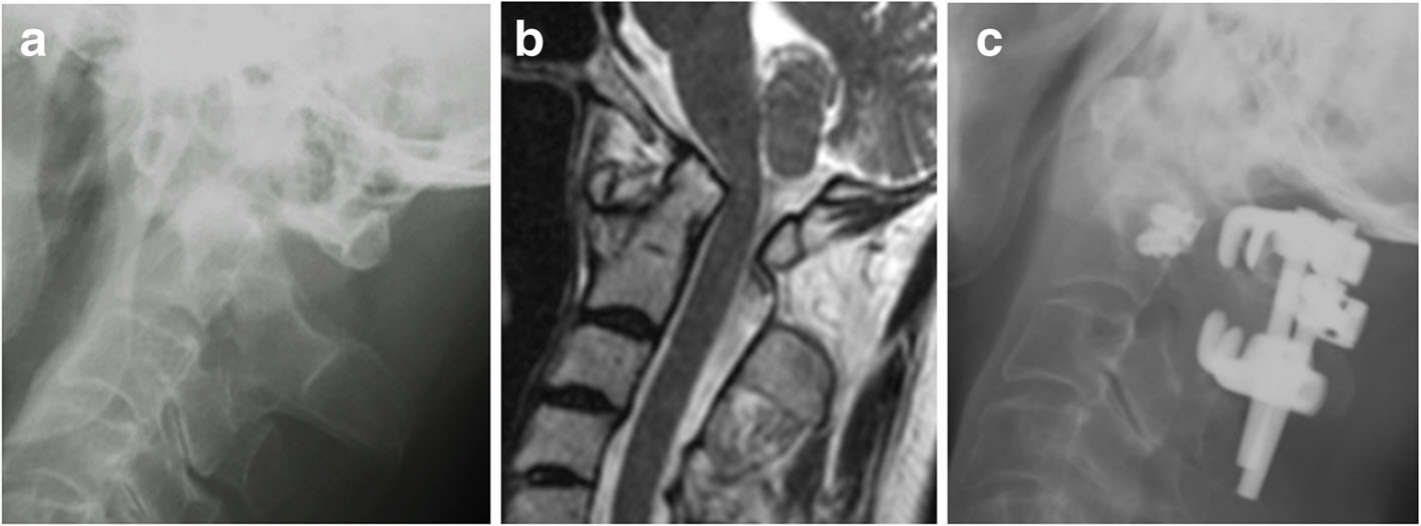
A 63-year-old female with rheumatoid arthritis and vertical subluxation. a Lateral view on a preoperative plain radiograph. The A1-A2 height was 30.9 mm. b Preoperative MRI. Vertical subluxation was noted. c Lateral view on a postoperative plain radiograph. The A1-A2 height had increased to 36.9 mm
